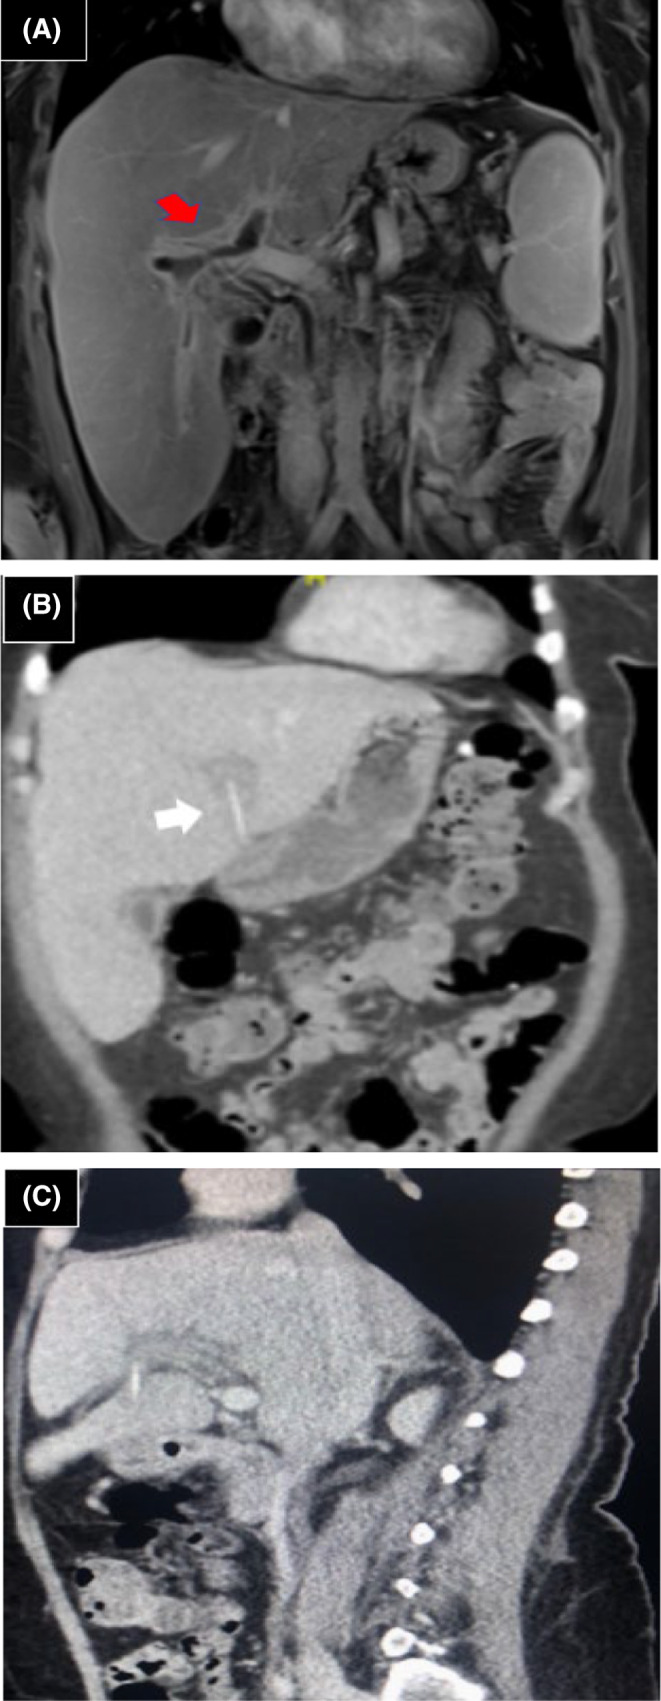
(A) Coronal (red arrow) MRI shows left, right, and upper main portal vein thrombophlebitis associated with inflammatory changes, (B) Coronal (white arrow) and (C) sagittal CT views demonstrated a 3.5 cm liner hyperdense structure.
